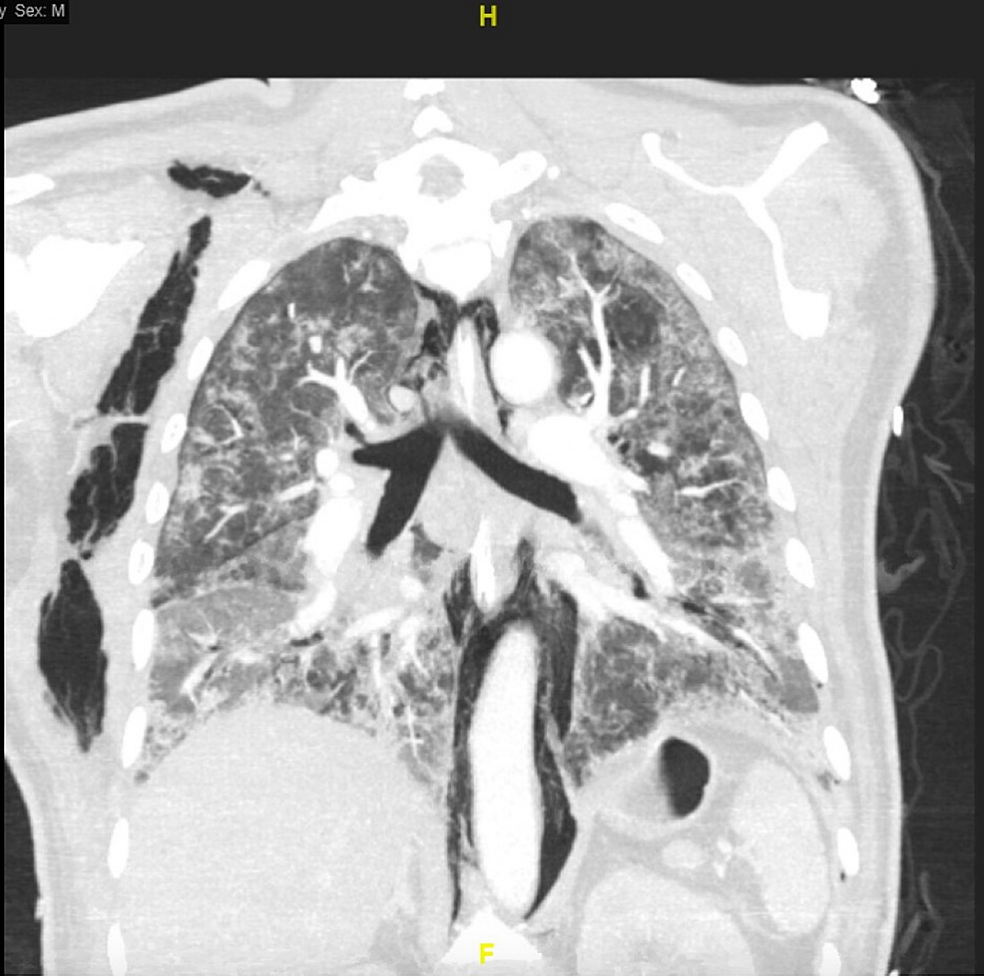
Coronal computed tomography (CT) showing extensive pneumomediastinum with air extending into the right supraclavicular region and the soft tissue of the right lateral chest wall
